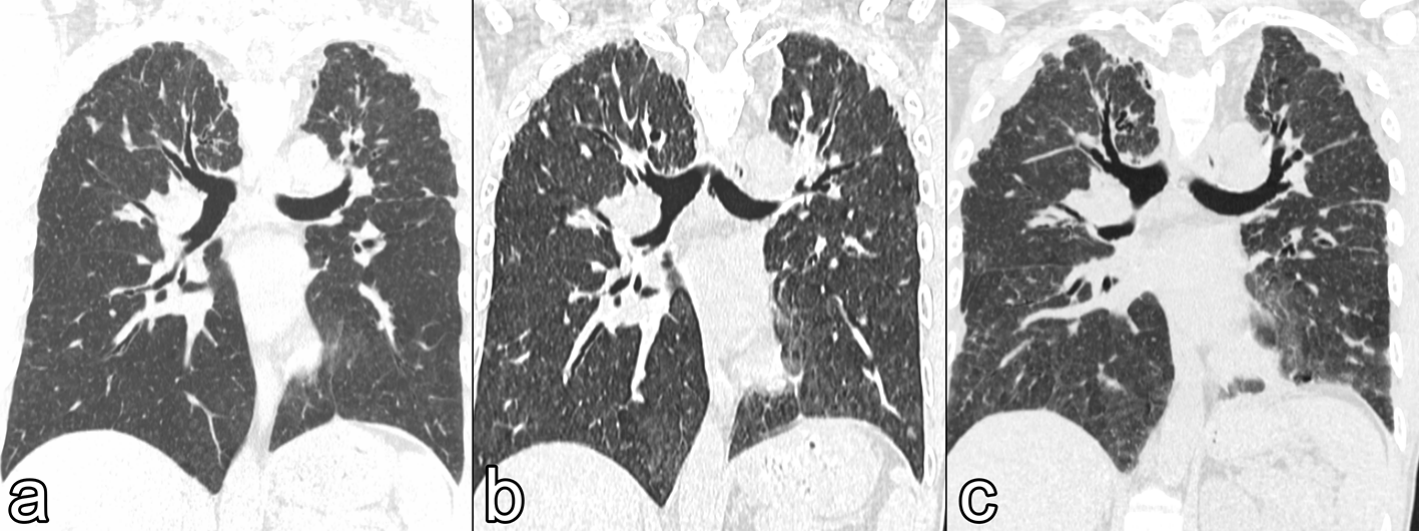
Coronal HRCT images showing the progression of PPFE on the native lung. (a) Shows mild bilateral apical pleural thickening with some subpleural reticulation. (B) After 1 year, HRCT shows a moderate increase of the irregular pleural thickening, together with traction bronchiectasis and early upper-lobe volume loss; at this stage, surgical lung biopsy confirmed the diagnosis of PPFE. (c) 2 years later, HRCT shows a further increase in irregular apical pleural thickening with subpleural reticulation and traction bronchiectasis; upper-lobe volume loss is more marked on the left lung. HRCT, high-resolution CT; PPFE, pleuroparenchymal fibroelastosis.
